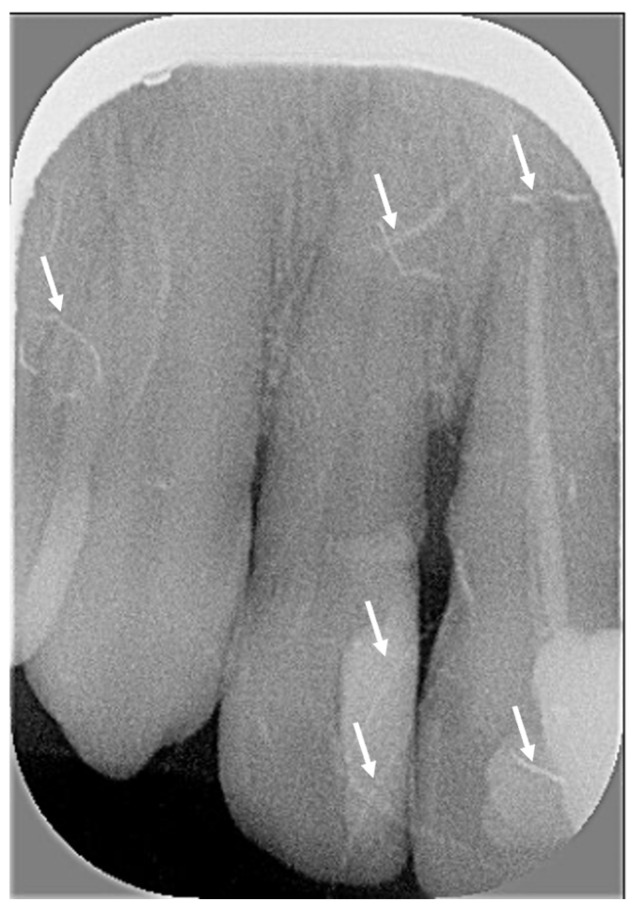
Grainy low-contrast X-ray with multiple radiopaque scratch artefacts (shown with white arrows).
